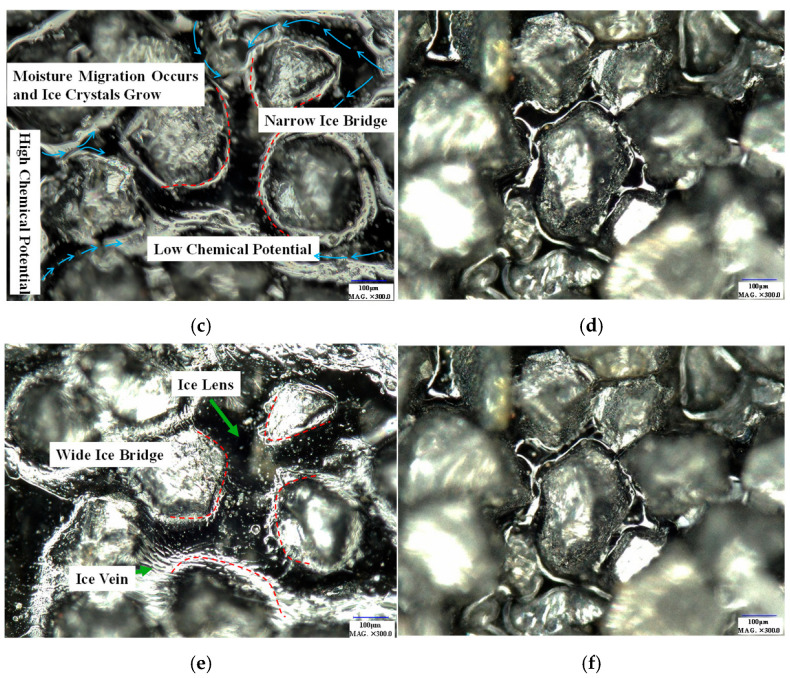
Comparison of phase transition in the low-temperature freezing region between conventionally and ultrasonically fabricated sand preforms with 4.5 wt.% moisture. (a) Initial water film morphology under conventional stirring. (b) Initial water film morphology under ultrasonic-assisted stirring. (c) Initial formation of ice lenses under conventional preparation. (d) Initial formation of ice lenses under ultrasonic-assisted preparation. (e) Full formation of ice lenses under conventional preparation. (f) Full formation of ice lenses under ultrasonic-assisted preparation.
